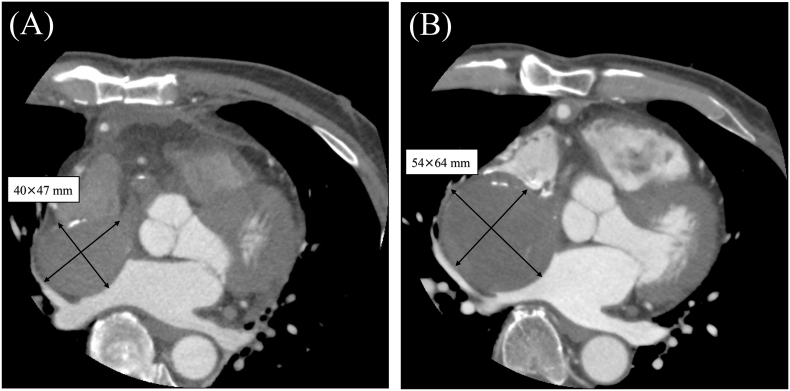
Contrast-enhanced chest computed tomography (CT). In the initial postoperative CT, the aneurysm had a diameter of 40 × 47 mm (A). However, a conspicuous aneurysm, measuring 54 × 64 mm in diameter (B), can be seen within the sinus of Valsalva and appears conspicuously devoid of any appreciable contrast enhancement. CT, computed tomography.
